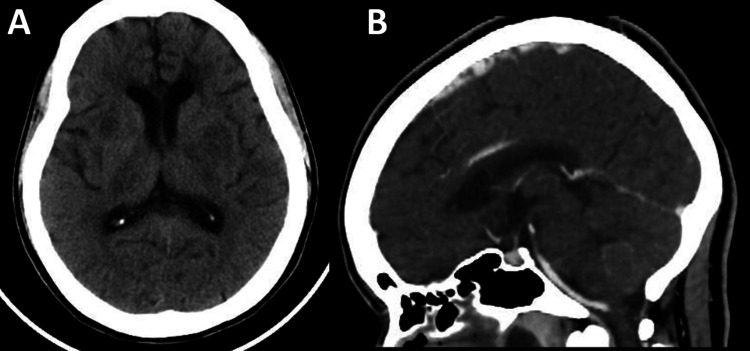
(A) Noncontrast brain computed tomography demonstrating no acute intracranial hemorrhage or mass effect. (B) Computed tomography cerebral venography demonstrating patent dural venous sinuses without evidence of venous sinus thrombosis
